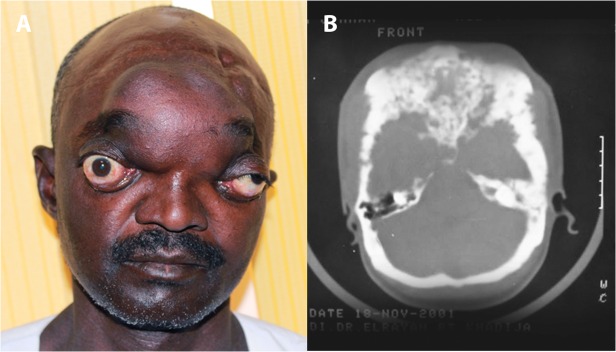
A: Showing massive head actinomycetoma with severe bilateral proptosis. This patient had vision loss in the right eye, and loss of hearing in both ears due massive intra-cranial involvement. B: Skull CT scan showing massive intracranial actinomycetoma involvement with bones destruction. The patient agreed to show his photos for publication purpose.
